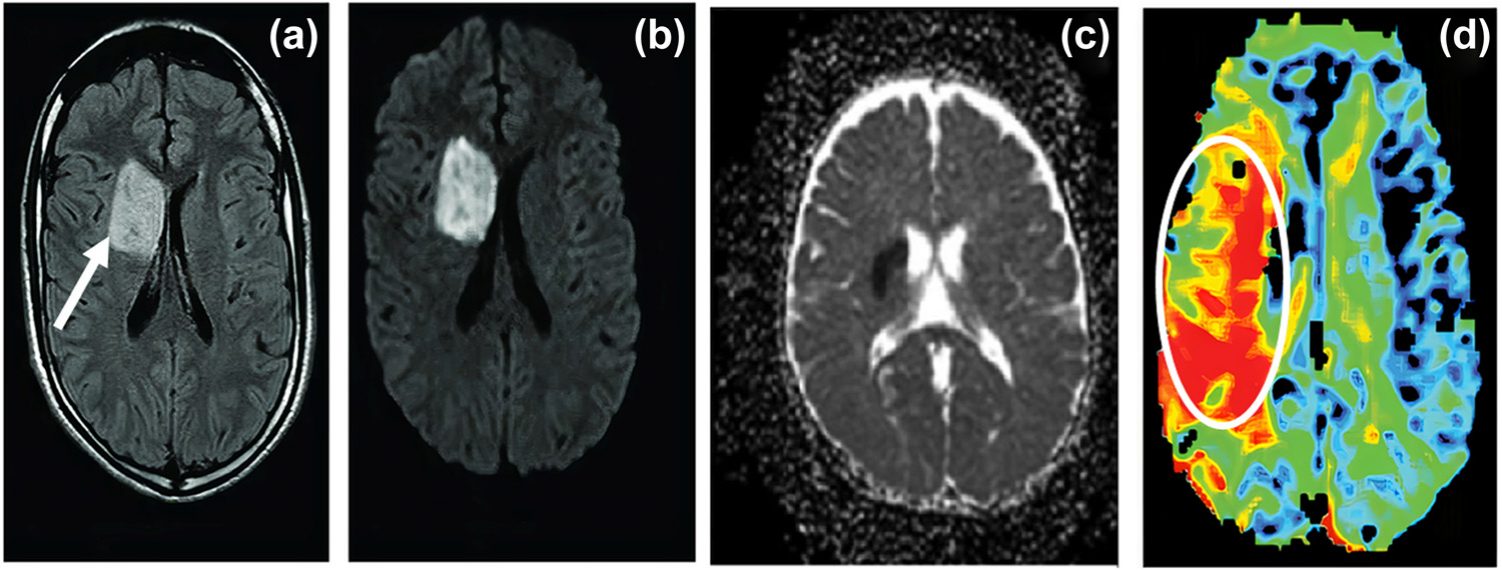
MRI on the brain. MRI on the brain has shown acute right basal ganglia infarct. (a) FLAIR, (b) DWI, (c) ADC, and (d) PWI.
